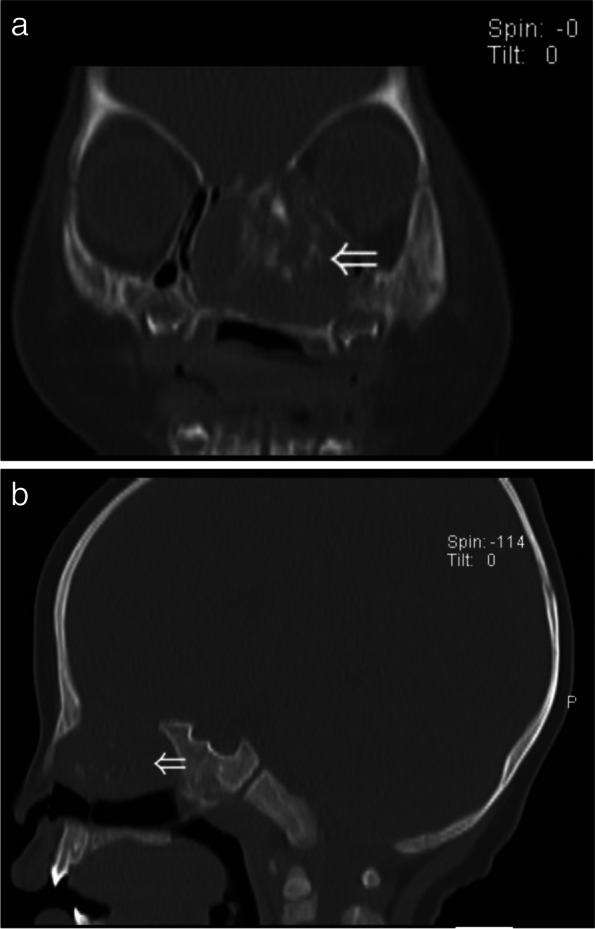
CT images. Coronal (a) sagittal (b) plane non-contrast images demonstrate a large soft-tissue density erosive mass occupying the left nasal cavity with extension into the left paranasal sinuses and occlusion of the nasopharnx. There is bony destruction of the anterior skull base and the left orbital wall along with multiple foci of calcification seen within the mass
